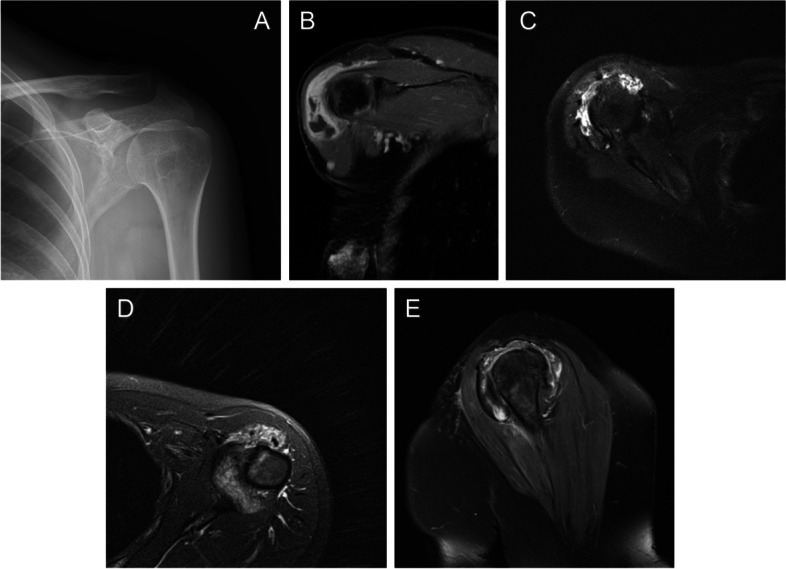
Radiological manifestations of shoulder PVNS. A Plain film shows low bone density in the front part of humeral head. B-E MRI shows low signal intensity related to hemosiderin increase in nodule content around shoulder cavity and high signal intensity related to the increase in fat tissue. PVNS, pigmented villonodular synovitis; MRI, magnetic resonance imaging
